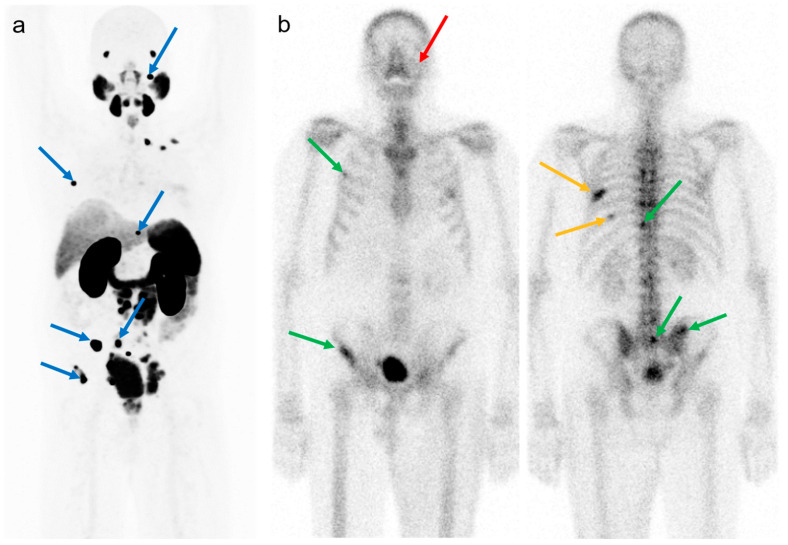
Example of comparison between [^68^Ga]Ga-PSMA-11 PET/CT and BS. (a) Coronal maximum intensity projection (MIP) of [^68^Ga]Ga-PSMA-11 PET/CT and (b) coronal projections of a BS with [^99m^Tc]Tc-DPD acquired 3 weeks after the PSMA PET. Lesions were selected on PSMA PET and labeled from cranial to caudal: the left mastoid, 3rd right rib, Th10, sacrum, right sacroiliac joint, and right iliac wing (blue arrows). All lesions but the left mastoid (red arrow) were labeled positive on the BS (green arrow). Of note, the intense uptake in the 7th and 9th ribs (orange arrow) on the left had corresponding fracture lines on PSMA PET with only minimal uptake.
